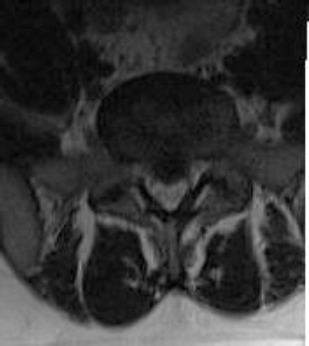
L5-S1 midline lumbar disc herniation in axial section MRI*.
